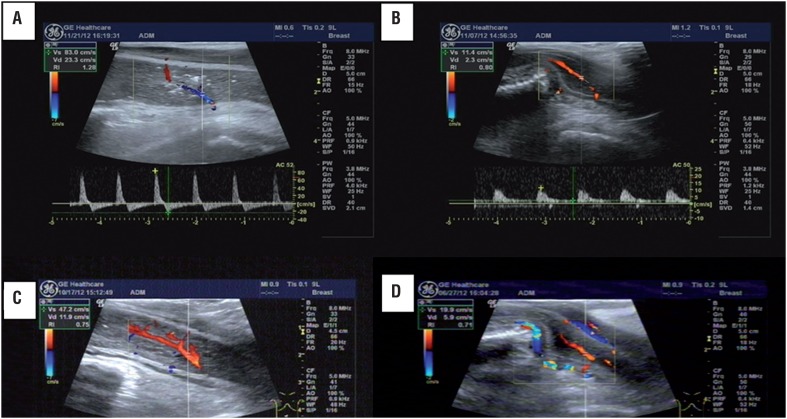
Abnormal arterial or venous responses to intracavernous injection of Bimix in ED patients. patients received a single intracavernous injection of Bimix (15 mg papaverine and 1 mg phentolamine) then ultrasonography was performed. (a) peak systolic velocity (psv)>35cm/sec and the resistance index (RI)=1 in a patient indicating a normal arterial and venous response. (b) In one ED patient psv<35cm/sec with the end-diastolic velocities (EDv)<5cm/sec suggesting abnormal arterial response. (c) in another ED patient psv>35cm/sec with EDv>5cm/sec indicating veno-occlusive dysfunction. (d) psv<35cm/sec with EDv>5cm/sec suggesting both arterial and venous abnormal responses.
